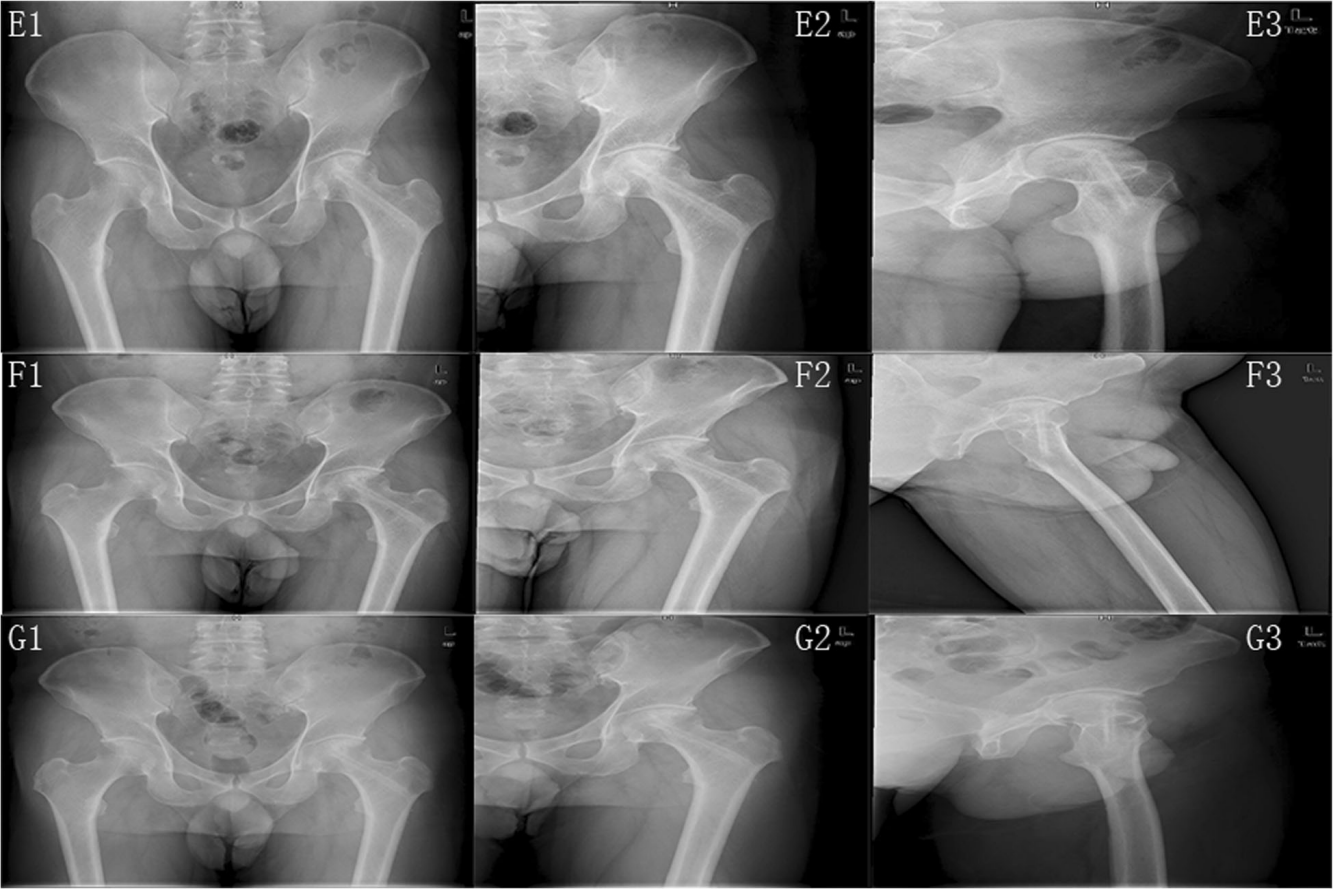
The radiographic outcomes of a 36 years old man with a long history of drinking and was diagnosed as left femoral head necrosis at Ficat II stage. A1–A5 The patient has early femoral head necrosis evaluated by X film and MRI respectively. B1–B3 The X film of 3 days after the operation. It shows that the femoral head is in normal shape and the grafted fibula integrates tightly in the channel. C, D The images at 1 and 4 months after operation respectively. E1–E3 The images at 2 years after the operation. F1–F3 The images at 5 years after the operation, which shows the stability of the articular cartilage and femoral head. G1–G3 The images at 8.2 years after the operation. The femoral head is not progressed and keeps in good shape. The left femoral head was in Ficat I stage and the Harris score was 90 at the last follow-up
